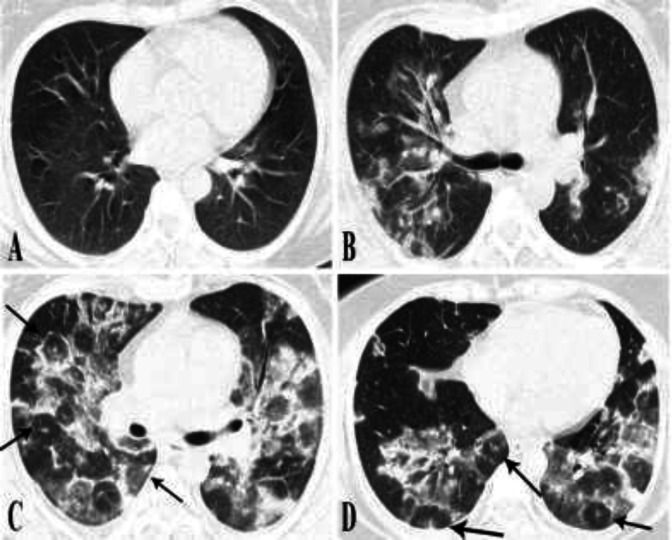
Serial imaging of case 1. The initial CT scan was normal (A). The on-admission CT scan taken after 8 days showed bilateral GGO with consolidative changes within (B). Ten days later, opacities increased in extension and density, with parenchymal band formation and several foci of target appearance (arrows) (C-D).
